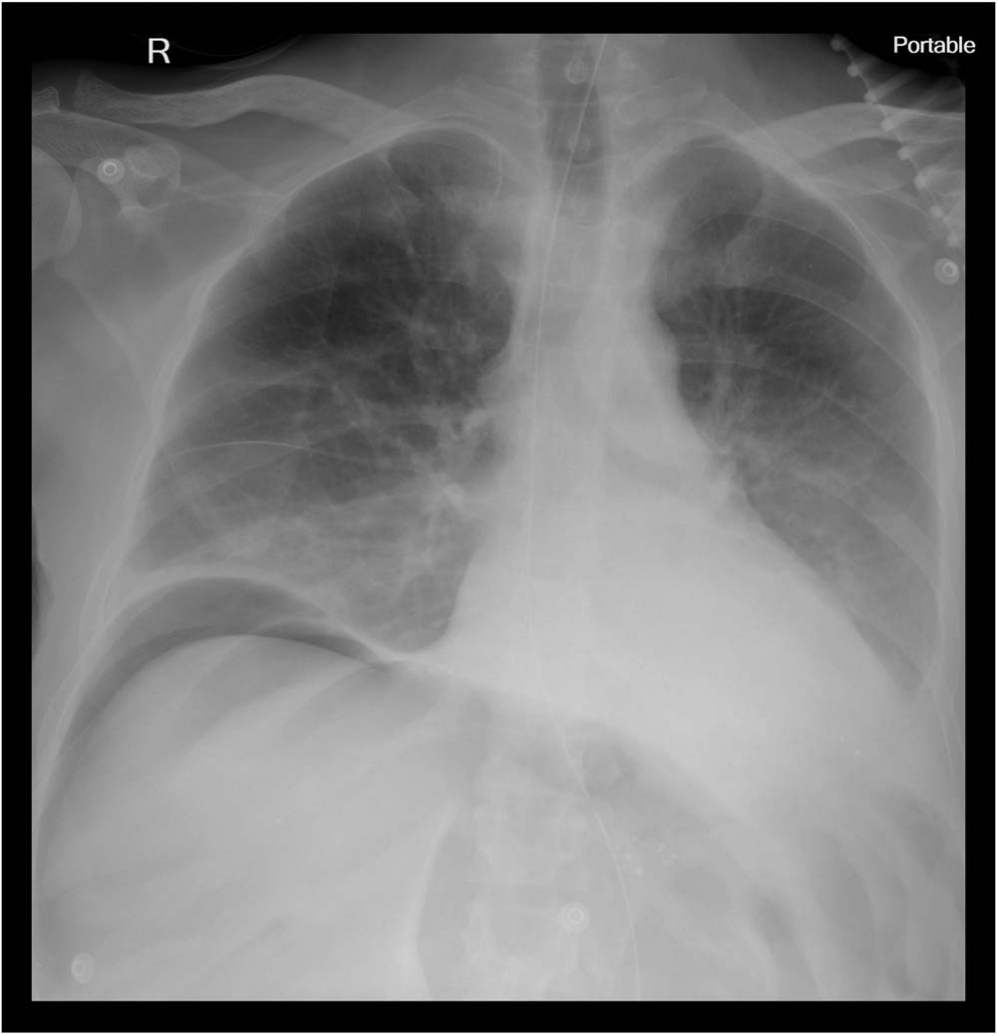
A portable chest X-ray with free air under diaphragm.
